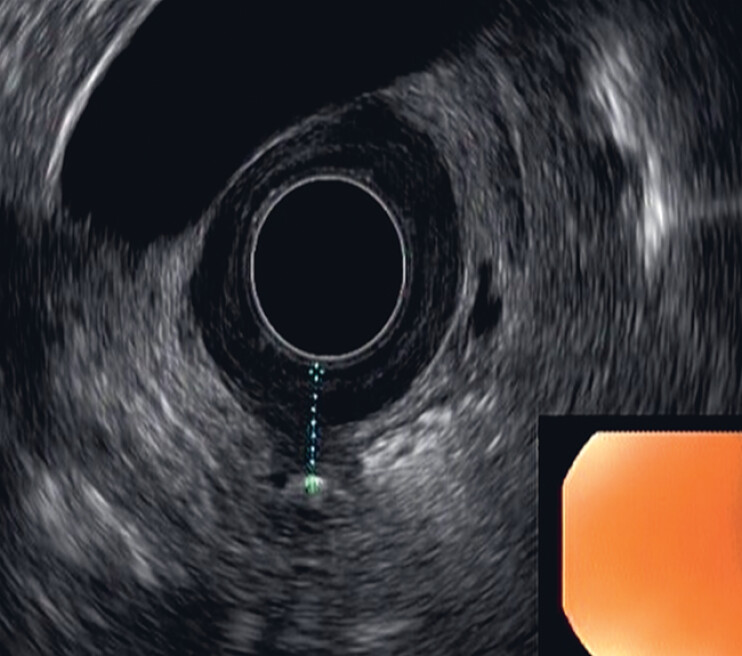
Ultrasound showing a strong echoic image outside the gastric wall at the site of the antral scar.
